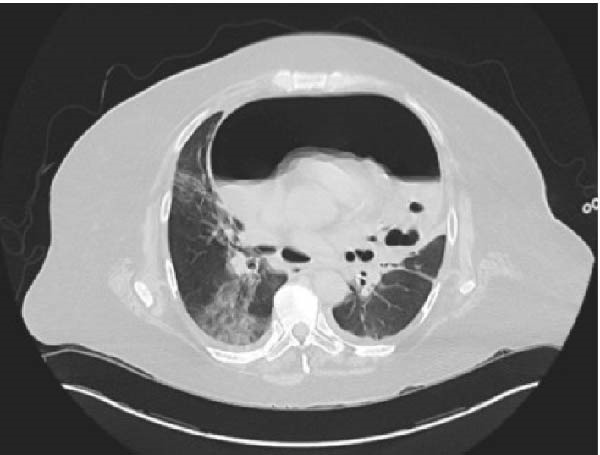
Transverse slice from CT imaging demonstrating large loculated hydropneumopericardium.
